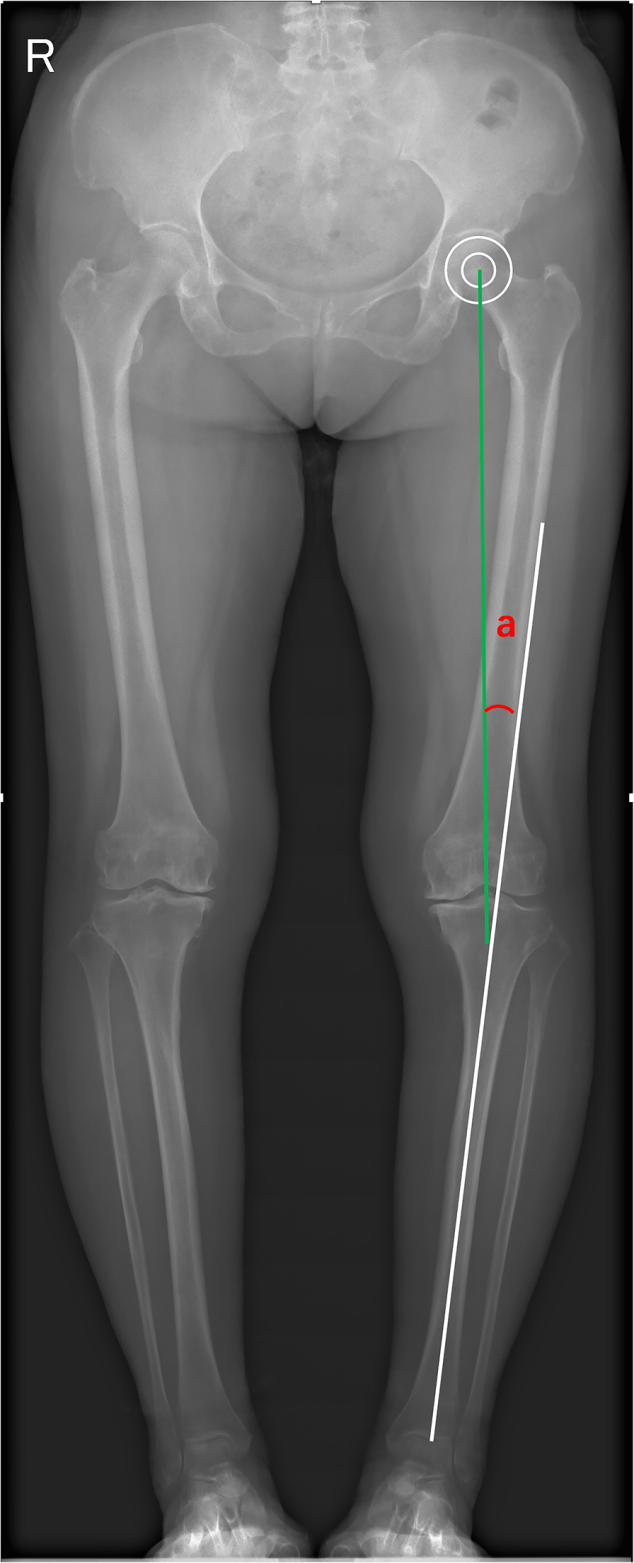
HKA angle measurement on a full-leg standing anteroposterior (AP) radiograph. HKA angle(a) was defined as the angle formed by the intersection of a line drawn from the center of the femoral head to the intercondylar fossa of the distal femur (green line) and a line drawn from the intercondylar spine of the proximal tibia to the center of the ankle joint (white line)
